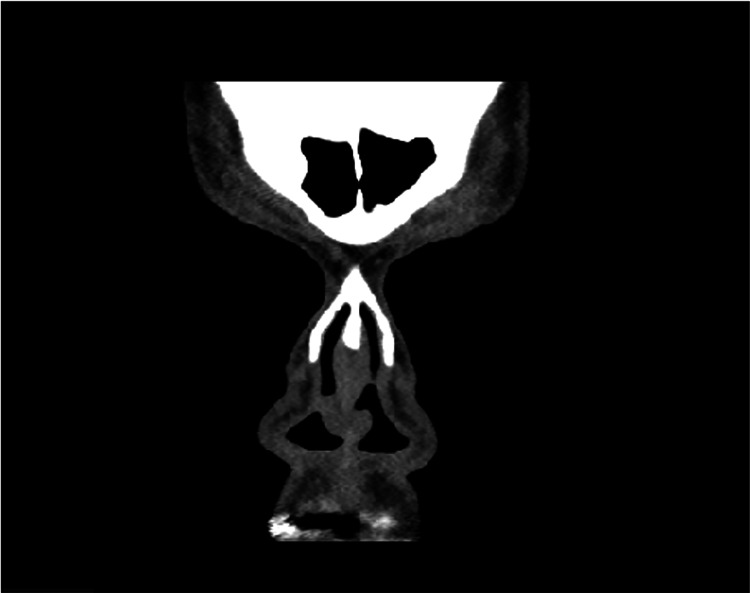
A computed tomography scan of nasal and paranasal sinuses shows an anterior cartilaginous nasal septal lesion.
